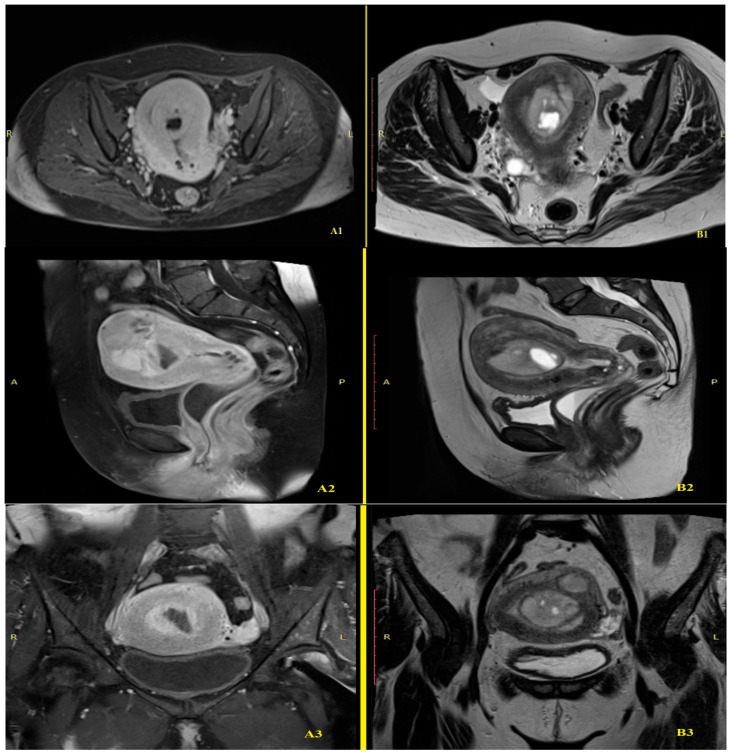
MRI comparison of T1-weighted post CIV: A, anterior; P, posterior; L, left; R, right; ((A1): transverse, (A2): sagittal, (A3): coronal) and T2-weighted ((B1): transverse, (B2): sagittal, (B3): coronal) sequences.
